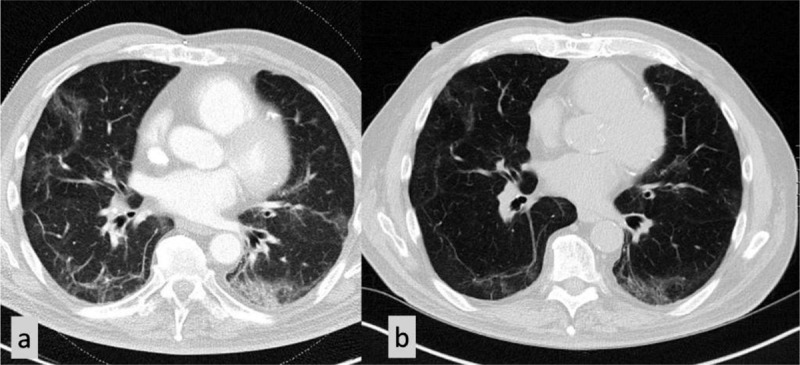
A 73-year-old man showed on covid-scan multiple areas of ground-glass opacities in both lungs but also a more reticular pattern with thickening of intralobular lines and irregular lines and bands (a). A control CT scan after 20 weeks showed the persistence of irregular lines and discrete signs of interstitial fibrosis (b).
